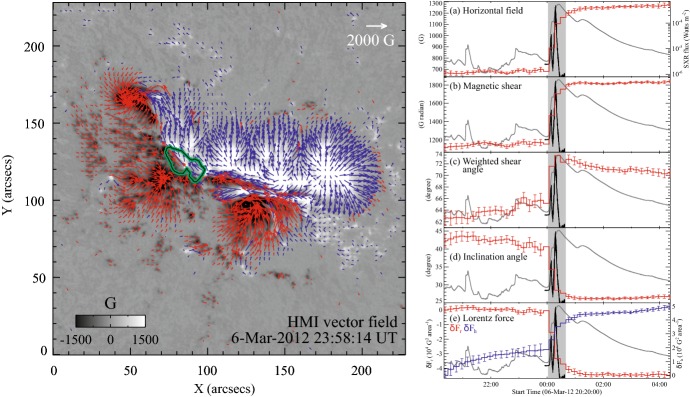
(Left) HMI vector magnetogram on 2012 March 7 showing the flare-productive AR NOAA 11429 right before the X5.4 flare. (Right) Temporal evolution of various magnetic properties of a compact region (green contour in the left panel) at the central PIL, in comparison with the light curves of GOES 1–8 Å soft X-ray flux (gray) and its derivative (black). Note that in panel d, the inclination is measured from horizontal direction. The shaded interval denotes the flare period in the GOES flux. Image reproduced by permission from Wang et al. (2012c), copyright by AAS
